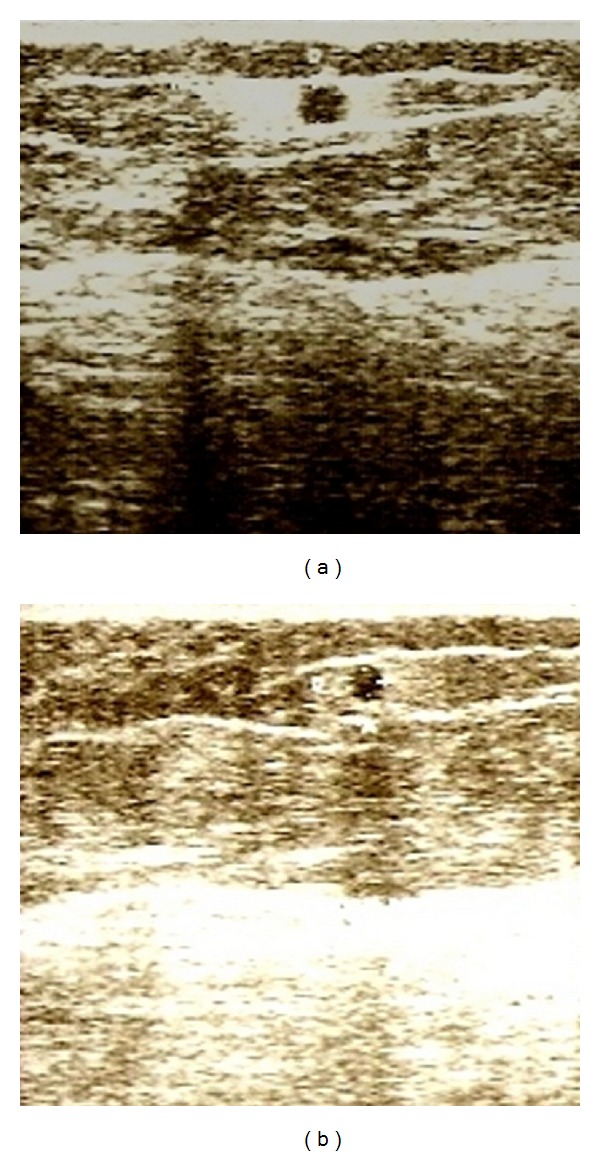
Ultrasonography before and after 10 sessions. These figures shows the presence of an adipose tissue graft performed 4 years before following lipoaspiration. The tissue is surrounded by interstitial fibrosis in (a), but after treatment (b) there is no visible fibrosis in this area.
